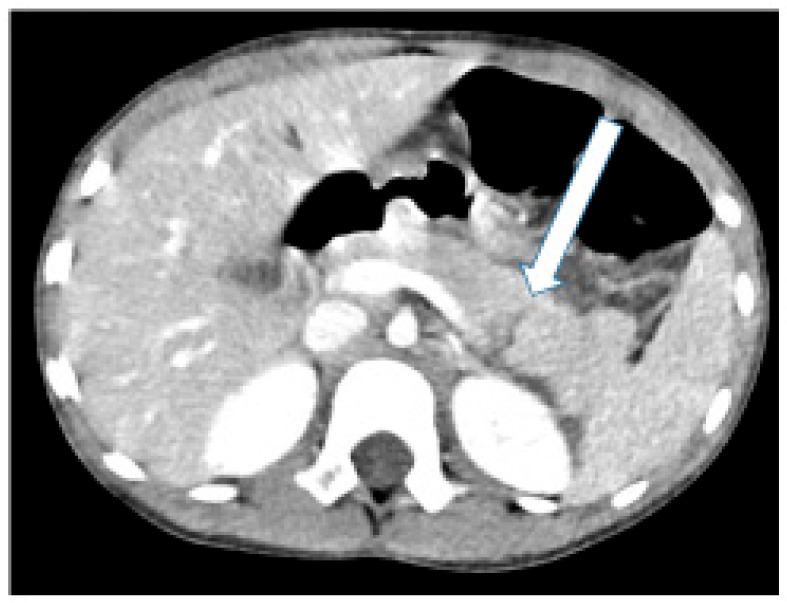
Abdominal computed tomography scan demonstrating a pancreatic injury to the left of the superior mesenteric vein (arrow).
